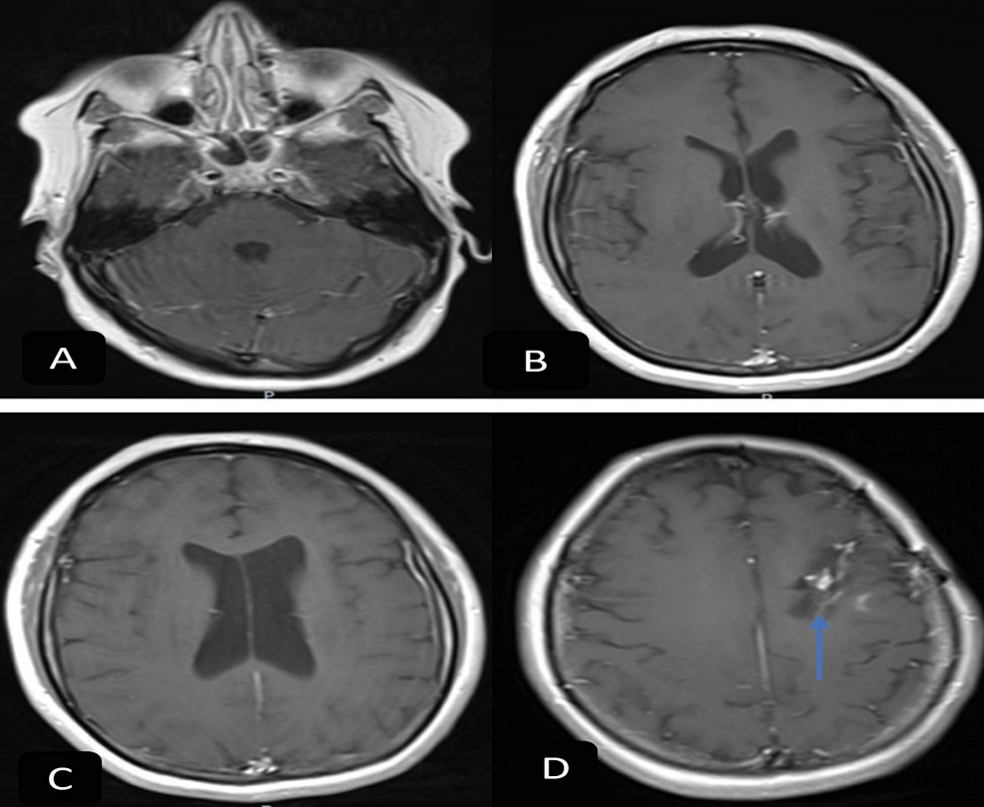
Brain MRI with contrast eight months after discharge T1 with contrast axial sequence showing resolution of multiple punctate enhancing lesions seen previously throughout the supratentorial and infratentorial regions (A-D). Expected progression of left frontal lobe hematoma (D, blue arrow).
